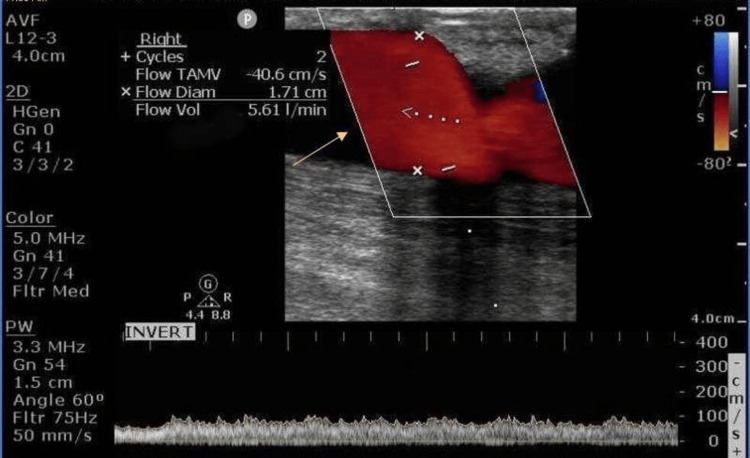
Duplex ultrasound The yellow arrow shows the blood flow in the left arm fistula
